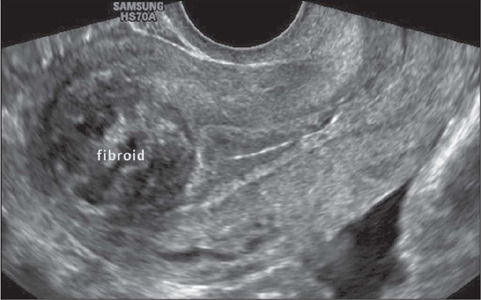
Transvaginal ultrasound image showing a submucosal uterine fibroid.
